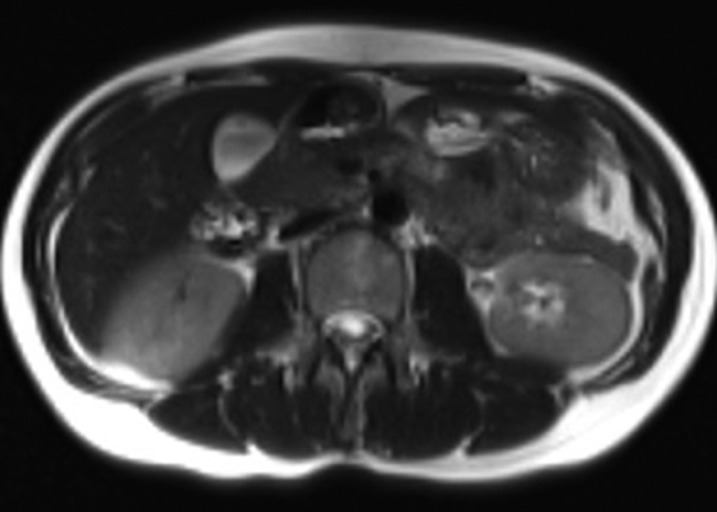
Abdominal magnetic resonance imaging revealed duodenal compression by the superior mesenteric artery, consistent with superior mesenteric artery syndrome.
